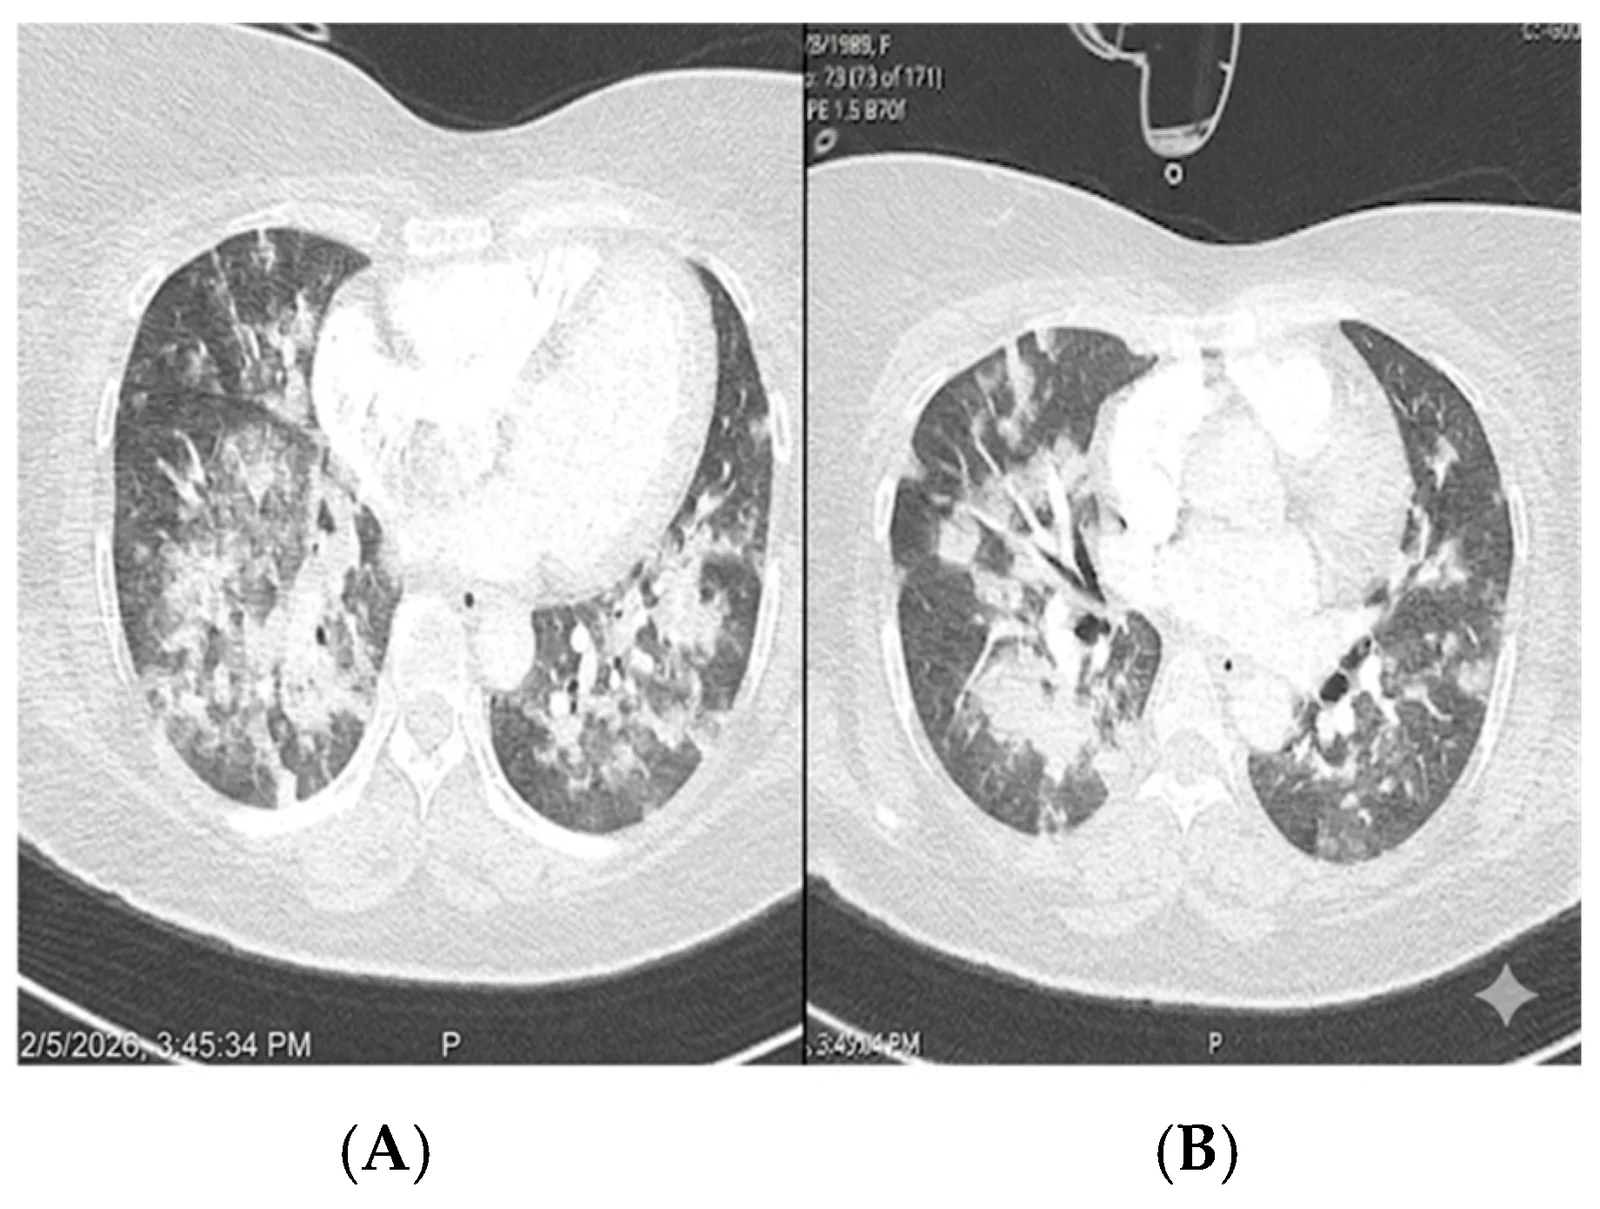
High-resolution chest CT scan (HRCT) slices. (A,B) Axial views demonstrate bilateral, multifocal peribronchovascular consolidations superimposed on widespread ground-glass opacities (GGO). Given the clinical history and acute development, these findings are highly suggestive of diffuse alveolar hemorrhage.
